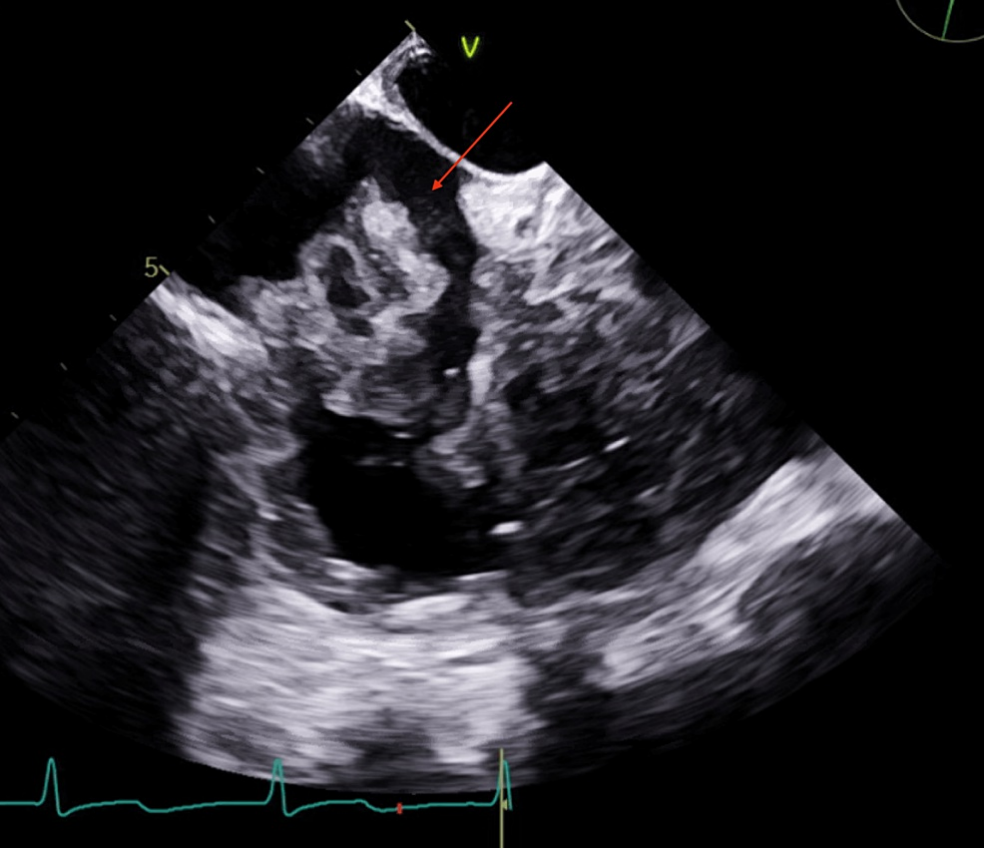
Transesophageal echocardiogram with intra right atrial mass with mobile attachments.
